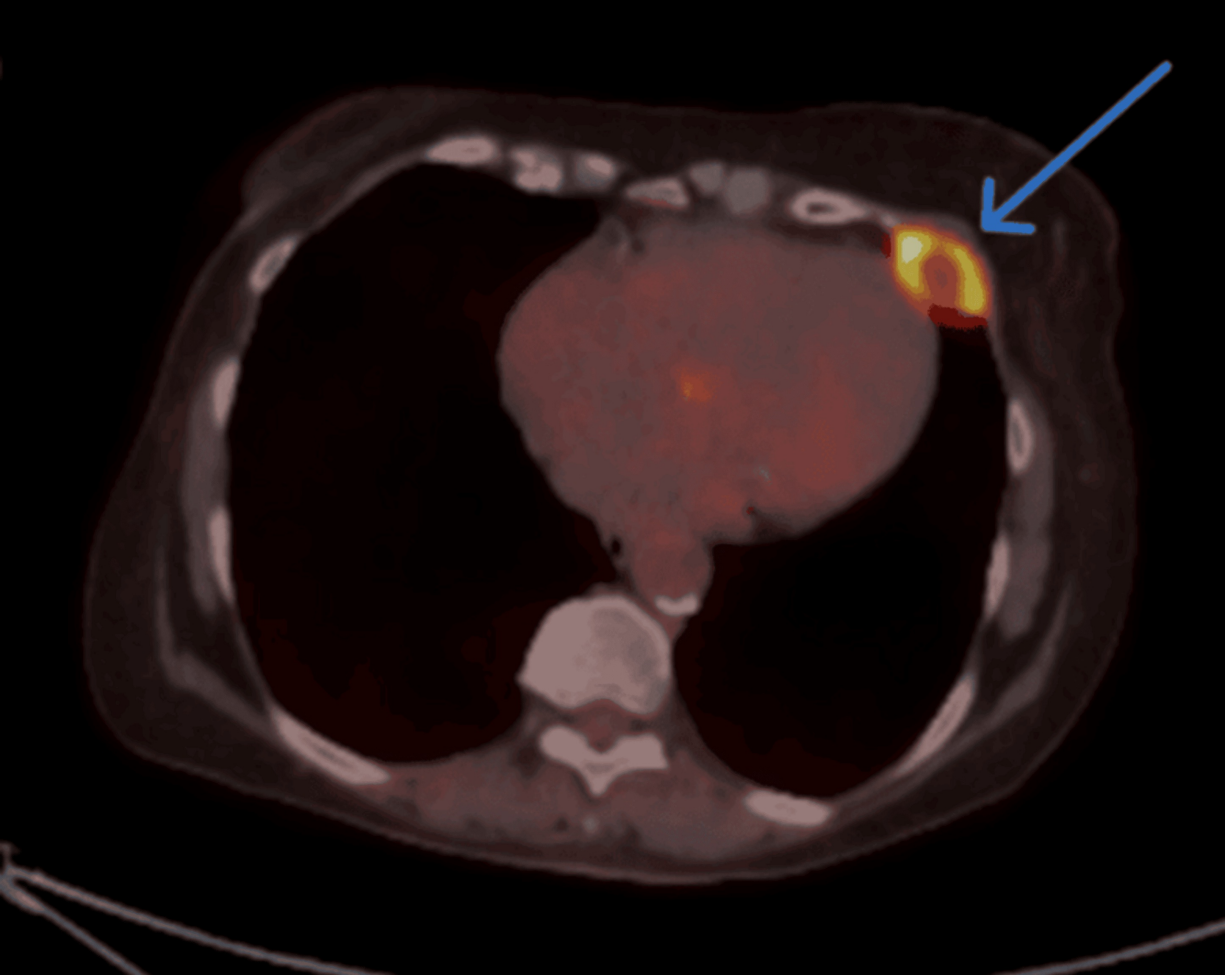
Axial PET-CT showing evolving necrosis in the metastatic rib lesion. Follow-up axial PET-CT of the chest demonstrated new areas of central necrosis within the fluorodeoxyglucose (FDG)-avid lytic lesion in the left anterior fifth rib, suggestive of a partial response to therapy.
